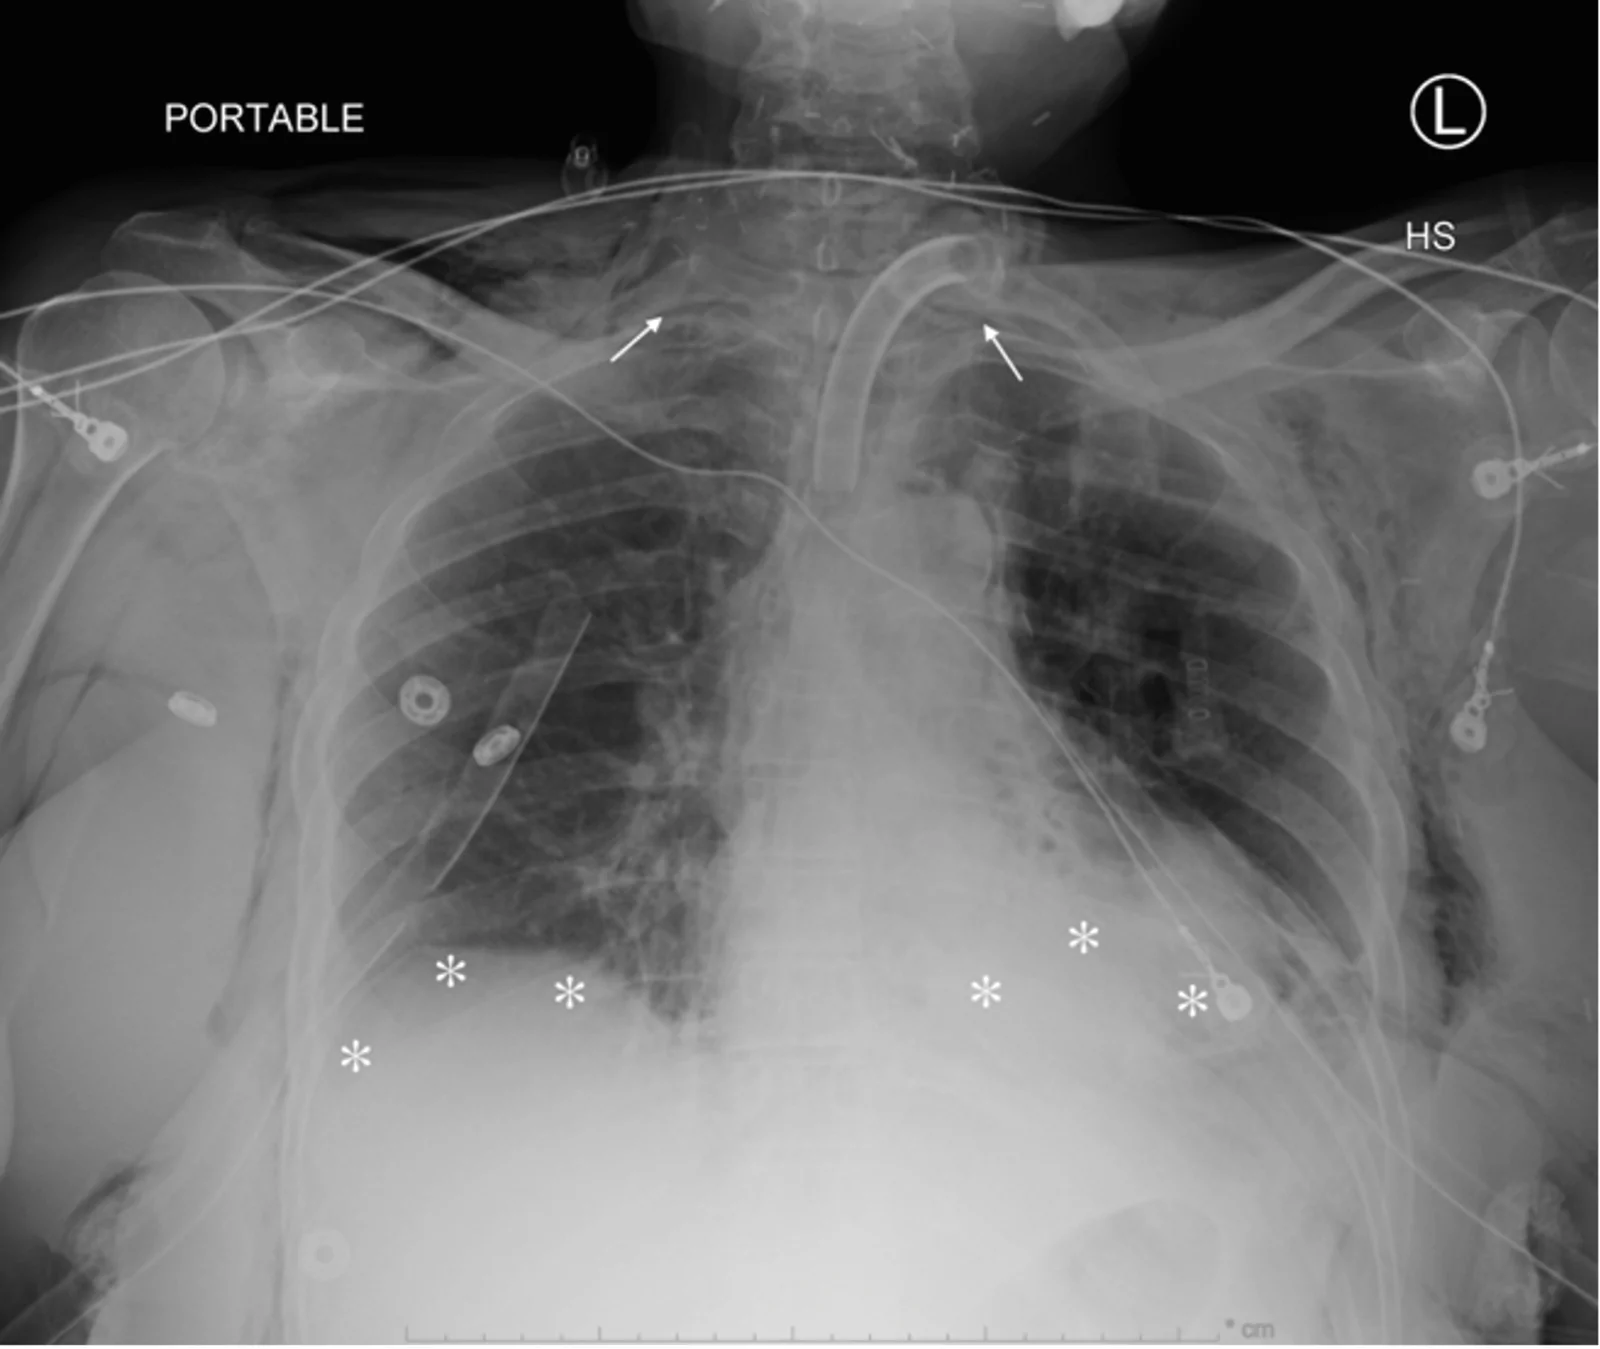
Follow-up chest radiograph Reveals resolved bilateral pneumothoraces with lung markings present in both superior lobes (white arrows) and resolved pneumoperitoneum, as the top of the diaphragm (white stars) is not surrounded by free air. Of note, the heart is no longer pushed upwards due to the pneumoperitoneum (Figure 1). HS: hora somni
